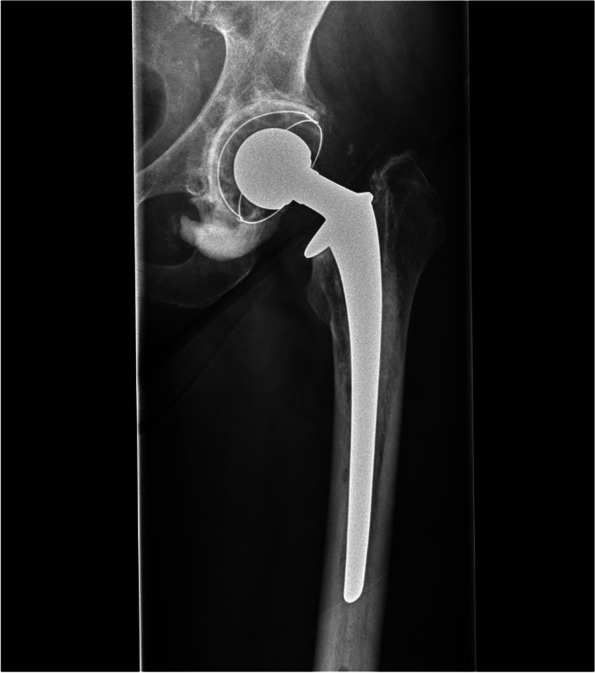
Total hip arthroplasty, anteroposterior radiograph. Postoperative radiograph after a total hip arthroplasty. Notice the cement extrusion at the acetabular side that caused the mechanical irritation of the psoas tendon
